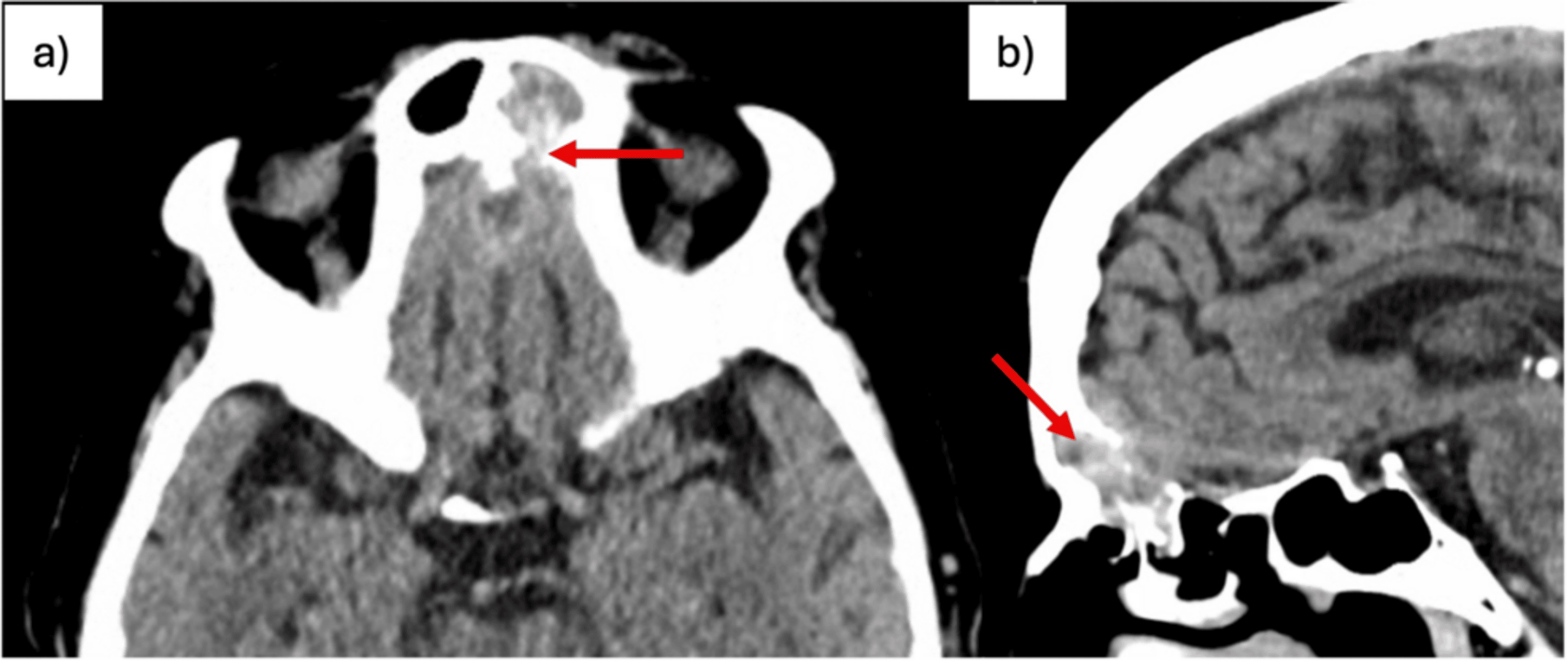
Cranial CT scan (a) Axial view; (b) Sagittal view. The left frontal sinus is filled with a soft-tissue component that, due to loss of the posterior bony contour, appears to extend anteriorly into the intracranial space along the midline (red arrows).
